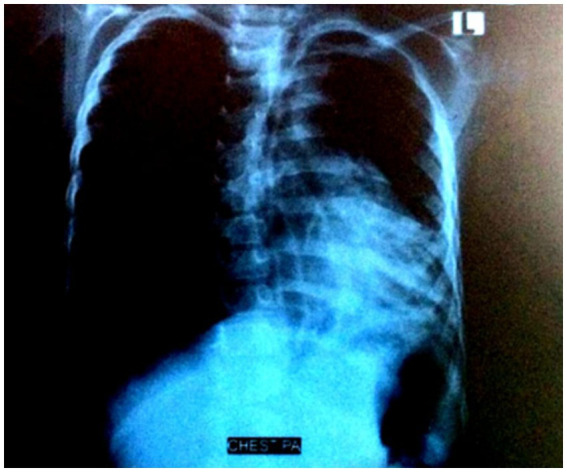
Chest X-ray on first day of admission showing right lung hyperinflation (pre-expulsion).
